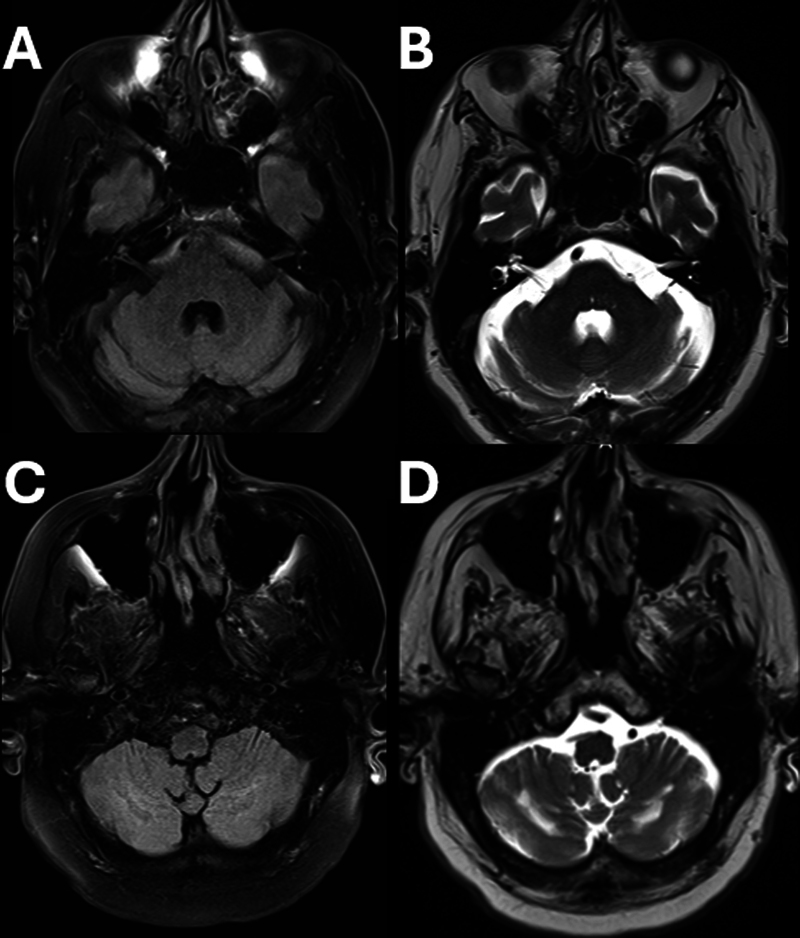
Four-month follow-up brain MRI scan. Axial FLAIR (
